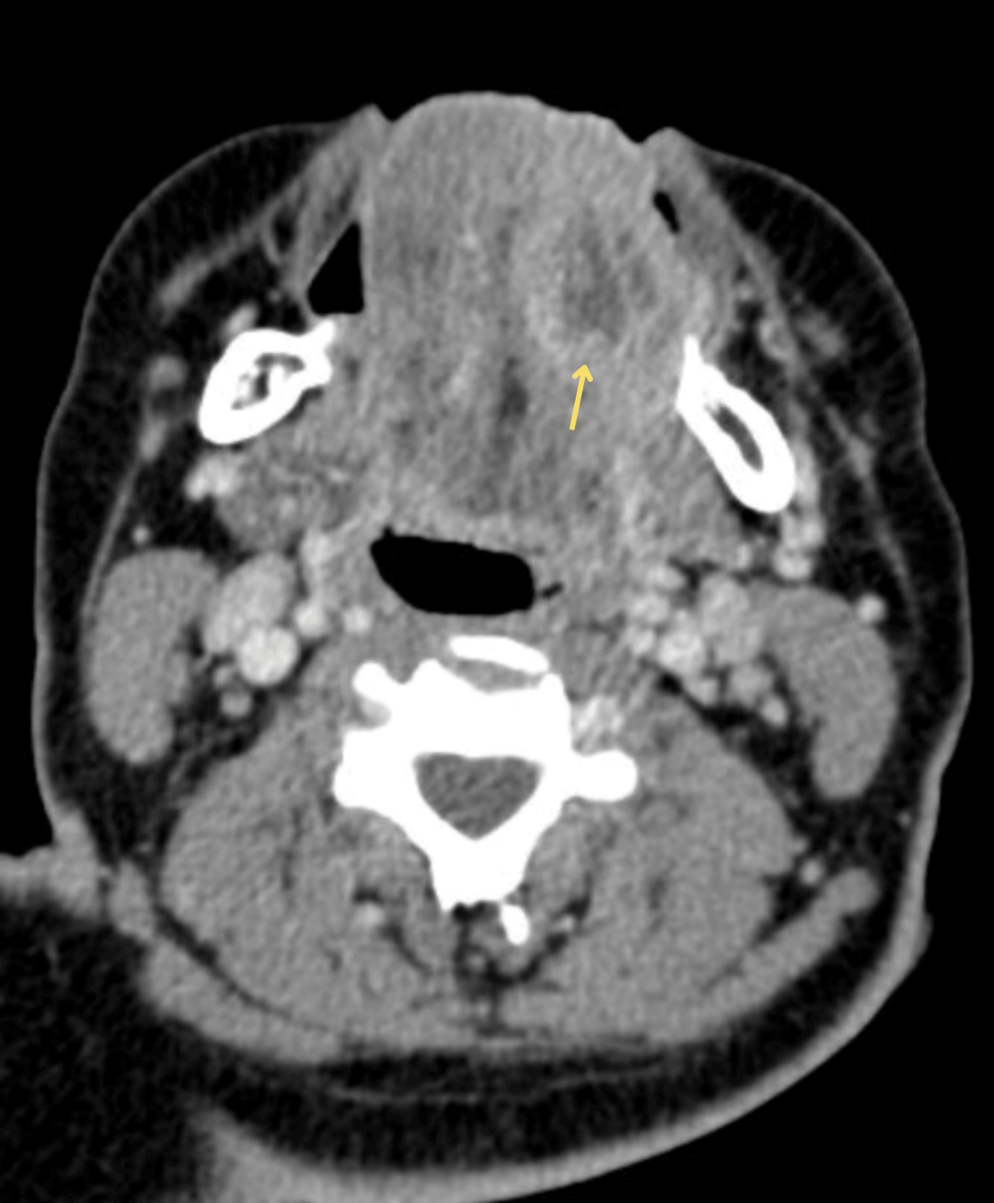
Contrast CT neck scan Abscess in the left hemitongue involving the intrinsic muscles (yellow arrow) shows collection with periferial enhancement.
